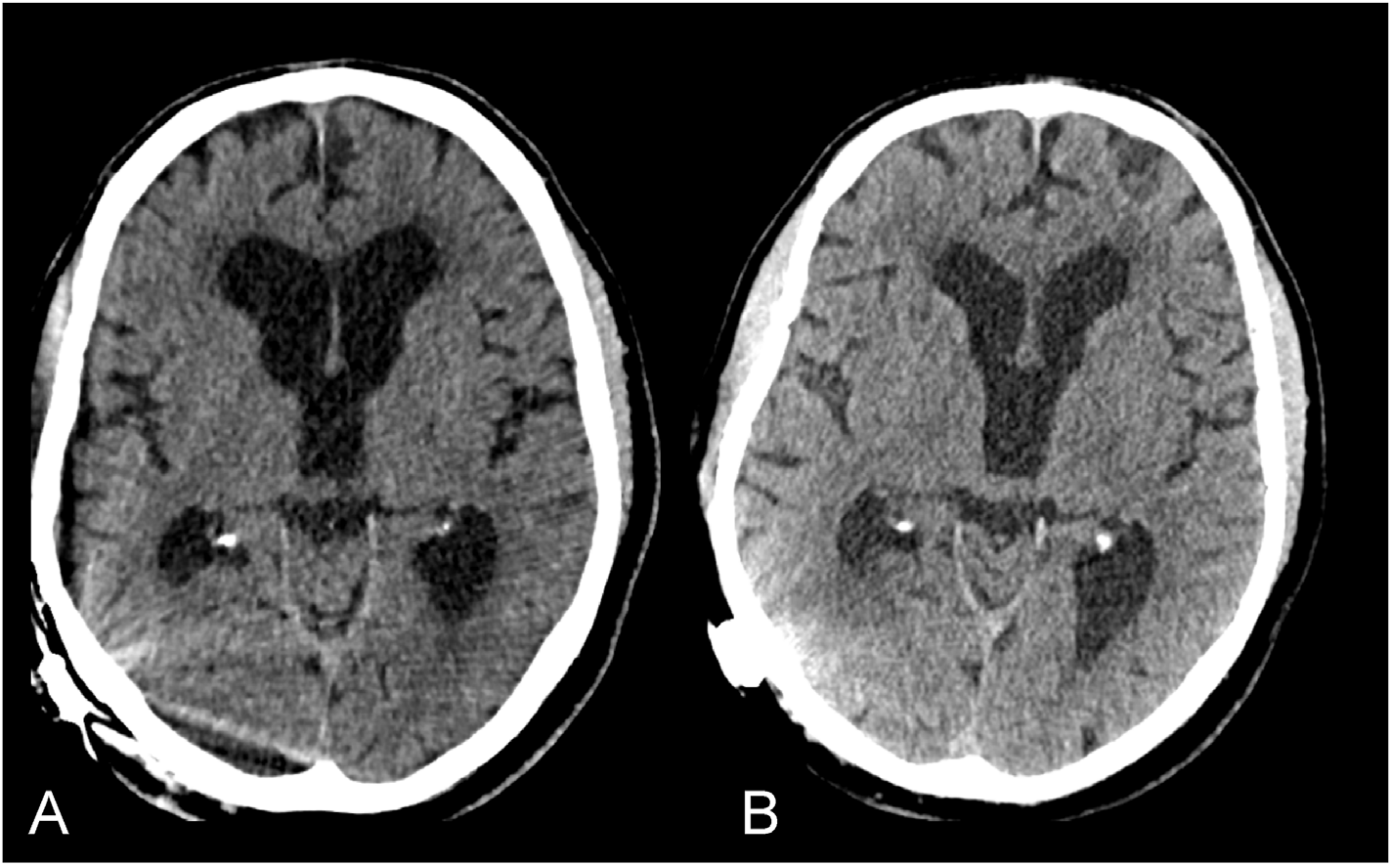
A, CT head immediately after insertion of the VPS. B, CT head upon presentation with hydrocoele, 4 months after VPS insertion, denoting unchanged ventricular calibre.
